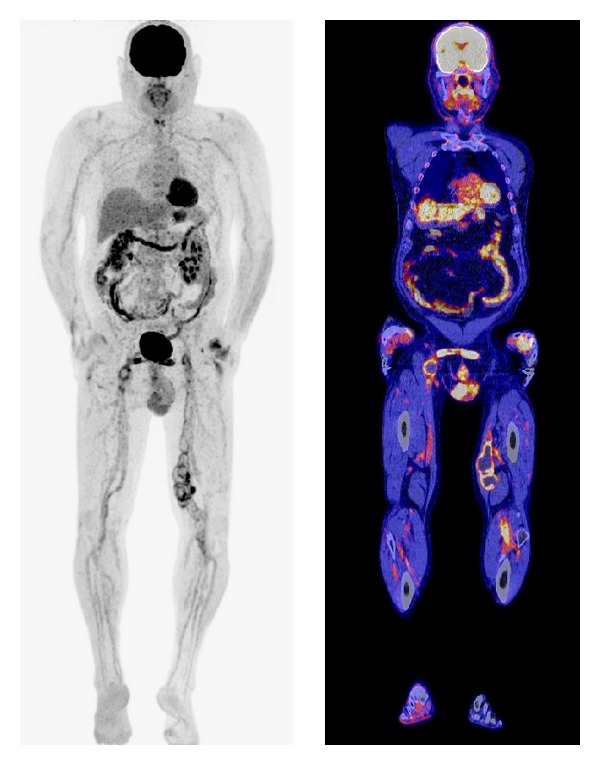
Coronal view of a fused ^18^F-FDG PET/CT image of a culture proven infected vascular prosthetic graft. In this particular case, a 70-year-old male patient underwent an ileofemoral bypass from the left to right side using Dacron. After 10 years the patient was readmitted with an occlusion of the bypass possibly due to a suture aneurysm. CT scan revealed an occlusion of the bypass with no signs of infection. ^18^F-FDG PET/CT scanning clearly showed increased FDG uptake at the level of the suture aneurysm. Ultimately,* Escherichia coli* were cultured from the graft.
